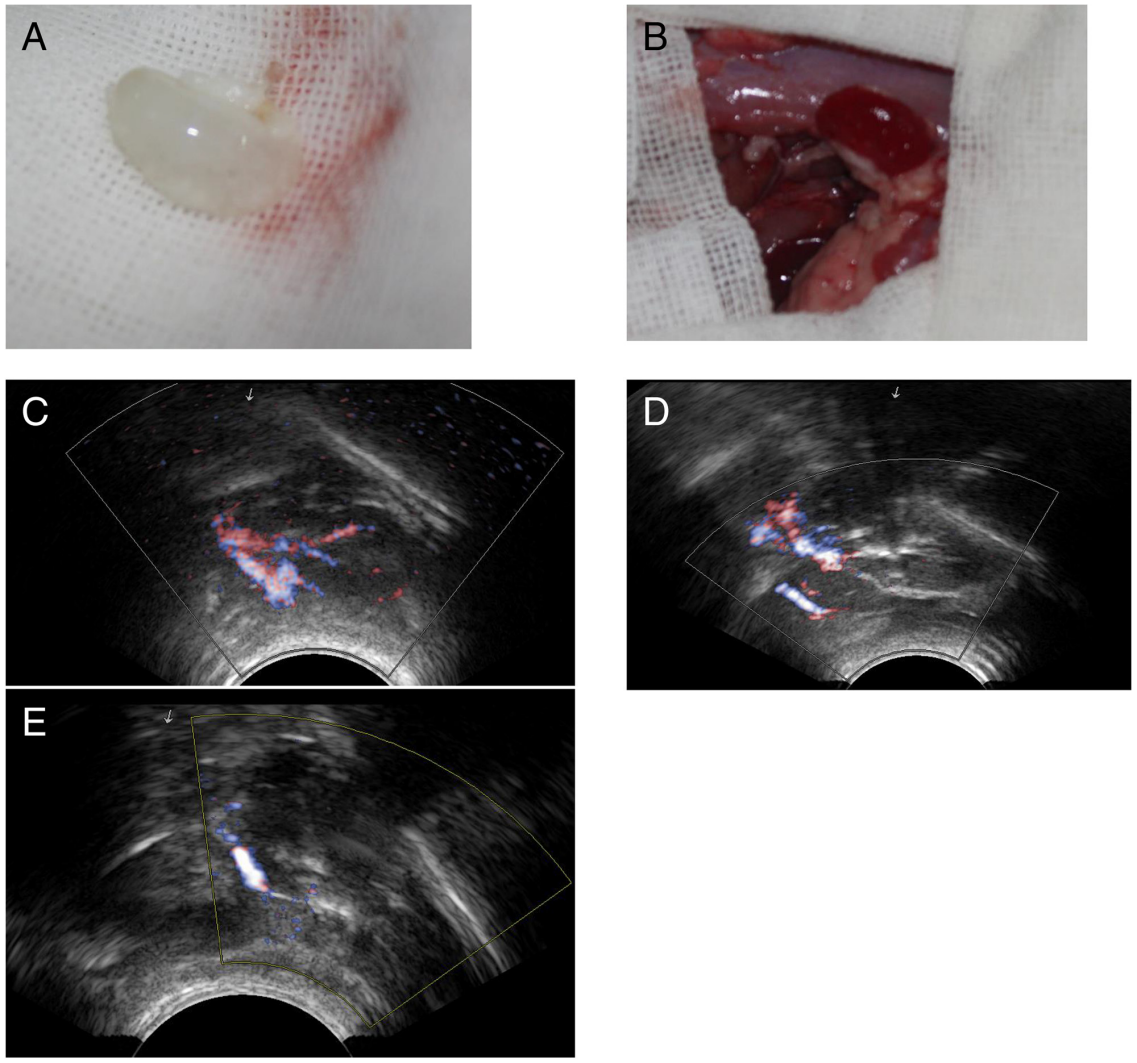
Implantation and explantation of the renal ECM scaffolds The gross appearance of the scaffold A&B. and the blood flow shown by B-scan ultrasonography C–E. (A), The acellular scaffold prior to implantation. (B), After removal of the clamps, blood flowed well within the whole scaffold. B-scan ultrasonography images of the implanted kidney scaffold obtained on day 2, weeks 1 and 2.
